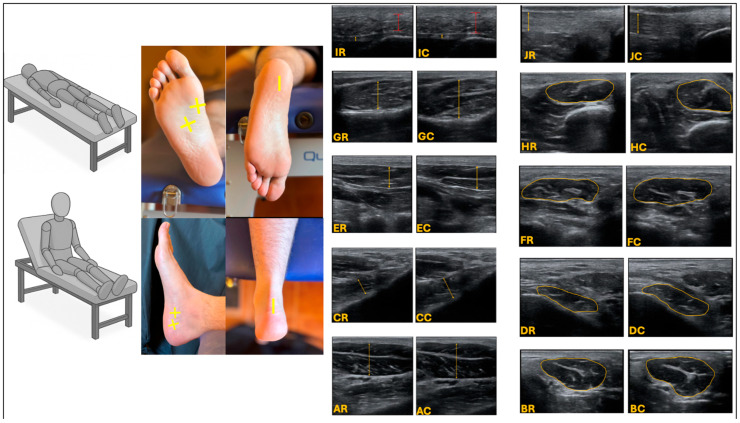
Ultrasound imaging thickness, and CSA for the AbH, FHB, FDB, QP, PF, CFP and AT. Abbreviation: AR, AbH thickness in resting state; AC, AbH thickness in contraction; BR, AbH CSA in resting state; BC, AbH CSA in contraction; CR, OP thickness in resting state: CC, OP thickness in contraction; DR, QP CSA in resting state; DC, QP CSA in contraction; ER, FDB thickness in resting state; EC, FDB thickness in contraction; FR, FDB CSA in resting state; FC, FDB CSA in contraction; GR, FHB thickness in resting state; GC, FHB thickness in contraction; HR, FHB CSA in resting state: HC, FHB CSA in contraction; IR. PF (orange colour) and CFP (red colour) thickness in resting state: IC, PF (orange colour), and CFP (red colour) thickness in contraction; JR, AT thickness in resting state; JC, AT thickness in contraction.
